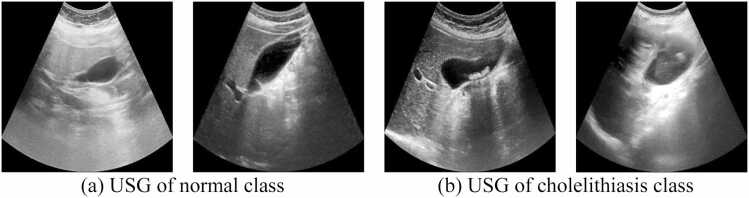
Sample ultrasound images used in experiments.
